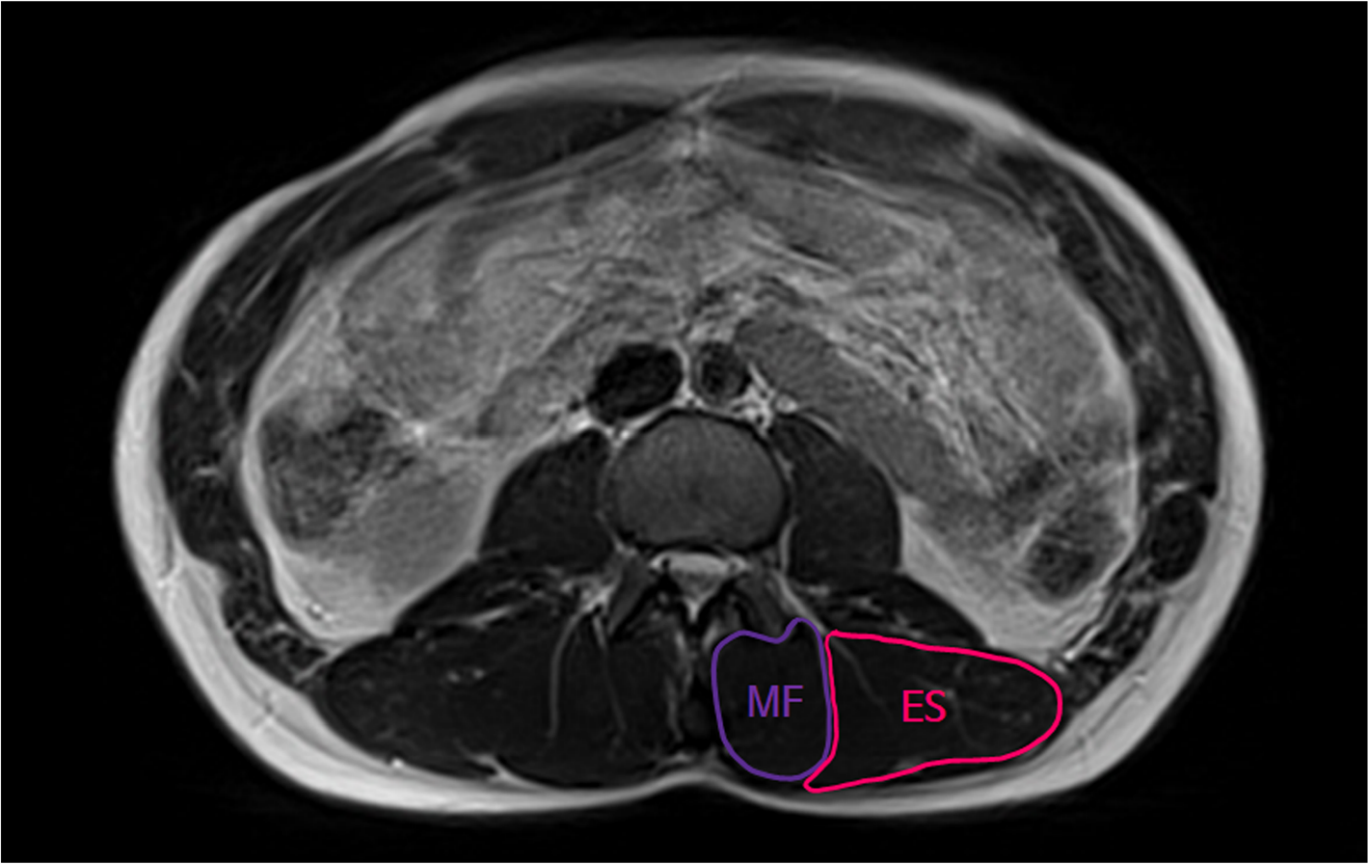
Magnetic resonance imaging of erector spinae and multifidus muscles at L4 vertebral endplate. ES = Erector spinae; MF = Multifidus
